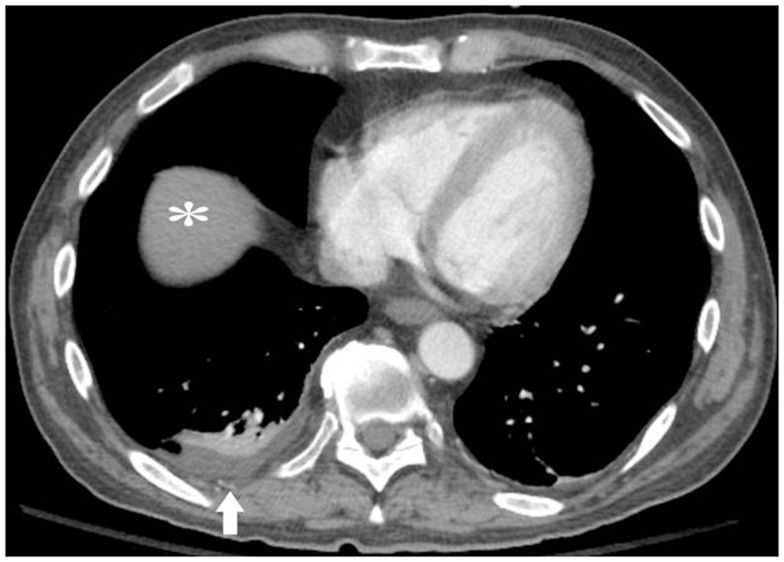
Post-treatment CT image after two cycles of fludarabine and cytarabine and four cycles of decitabine alone. White arrow = residual small right pleural effusion. No left pleural effusion or pericardial effusion. White asterisk = liver.
